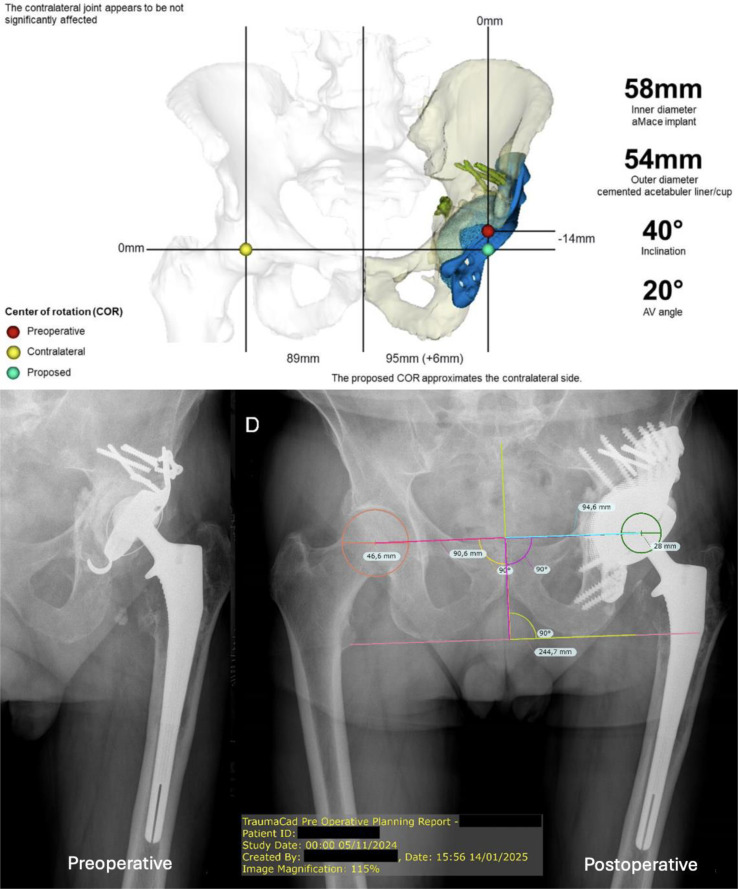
Complete case with 3D planification (Materialise) and radiographic measurement of *x*-axis and *y*-axis deviation of COR (case 10).
